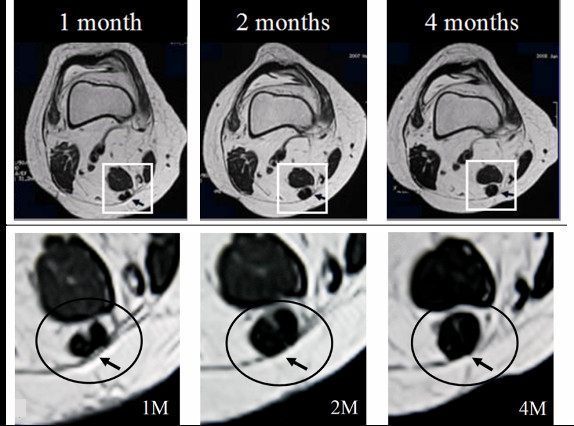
The changes observed on consecutive MRI at the level of the superior pole of the patella. MRI performed at 1, 2 and 4 months after the operation (a). The two round regions of low intensity were enlarged and connected by 2 months, and were unified by 4 months after the operation (b).
